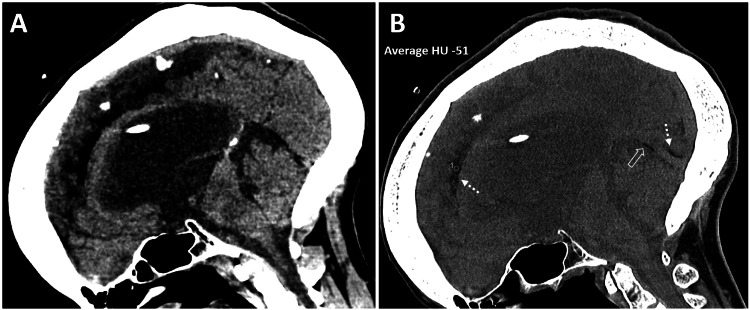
Sagittal CT depiction of supratentorial hydrocephalus, intraventricular hemorrhage, and dural fat deposition in chronic VP shunting. A 46-year-old female presented with altered mental status, in the setting of multiple VP shunt placements for obstructive hydrocephalus. An unenhanced sagittal reformatted CT image of the brain in (A) soft tissue algorithm and (B) bone algorithm with a soft tissue window setting shows marked supratentorial hydrocephalus with ventricular shunts and external ventricular drain catheters, along with intraventricular hemorrhage in the third ventricle. Incidentally, there is mild diffuse fat density (Hounsfield Unit -51) along the dura in the interhemispheric falx cerebri (dashed white arrows in B) and along the inferior sagittal sinus (open white arrow in B), best depicted in the bone algorithm with a soft tissue window setting. There is associated calvarial hyperostosis from chronic venous shunting. CT, computed tomography; VP, ventriculoperitoneal
